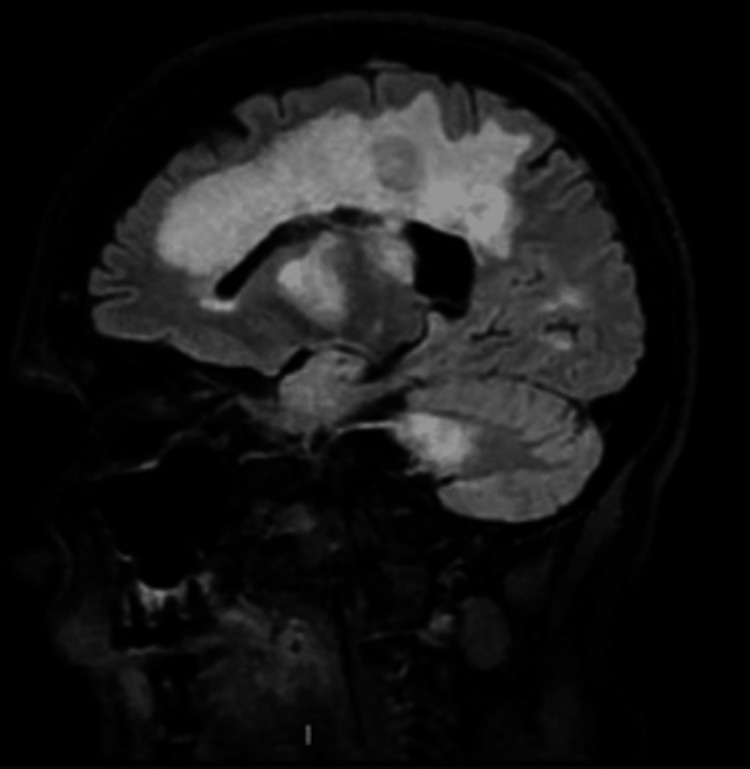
Sagittal view of MRI T2 FLAIR sequence of the lesions FLAIR: fluid-attenuated inversion recovery
